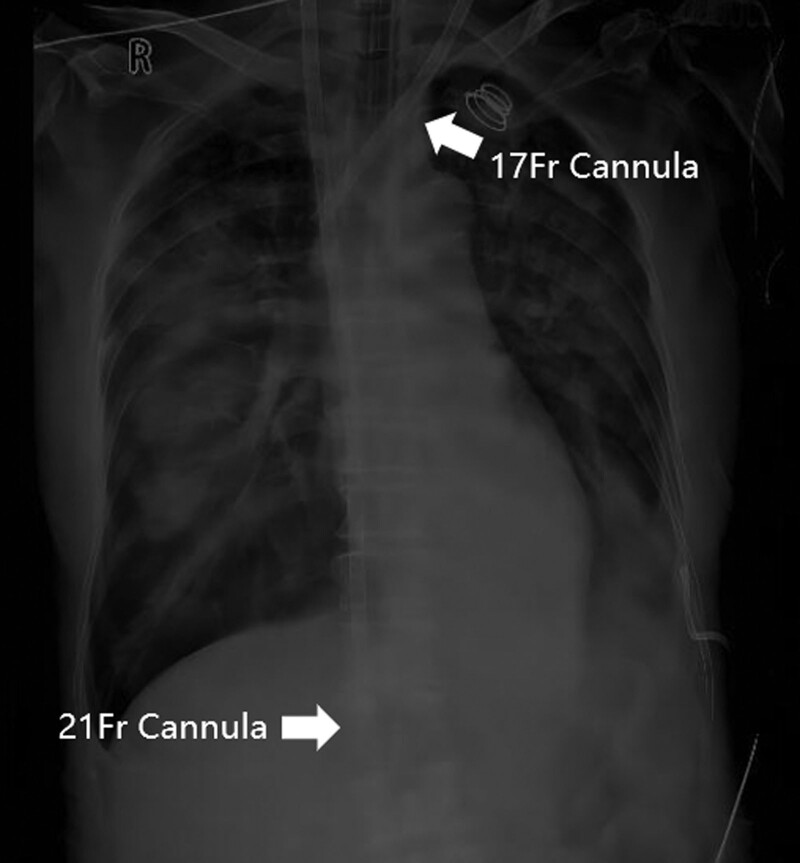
Ideal positions of the cannulas upon routine chest radiograph taken after second cannulation.
